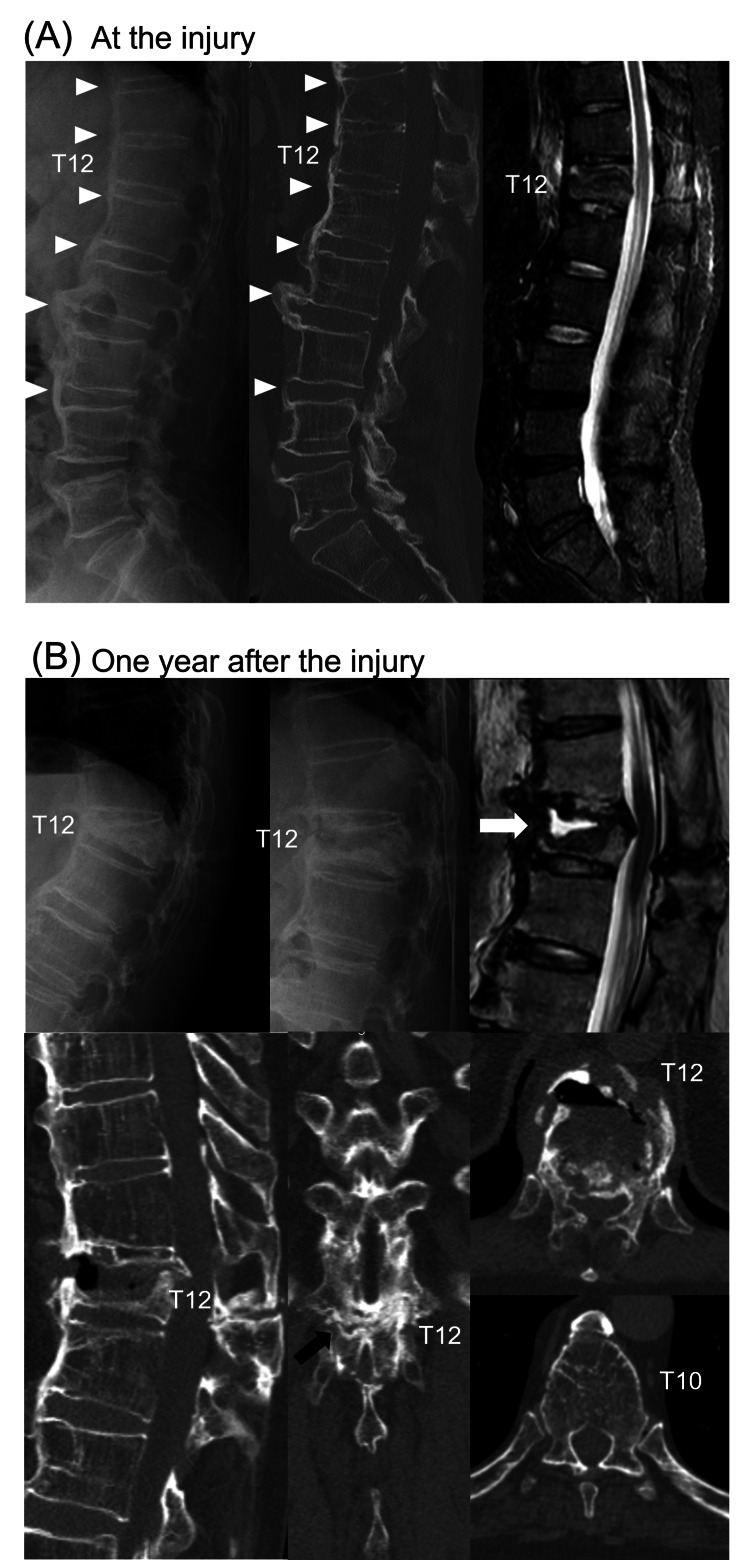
Preoperative radiological findings. The images show (A) radiological findings at the injury. T12 vertebral fracture was not diagnosed by x-ray (left) or CT (center), but by MRI (right). Ossification by DISH was observed between the thoracic segments and L4 (triangle). (B) Radiological findings after one year from the injury. X-rays showed inter-vertebral instability of T12 between the standing position (upper left) and the supine position (upper center). MRI showed a fluid collection at T12 (upper left, white arrow). CT images showed an anterior huge intervertebral cleft and transverse lamina fracture at T12 (black arrow). DISH: diffuse idiopathic skeletal hyperostosis; CT: computed tomography; MRI: magnetic resonance imaging
